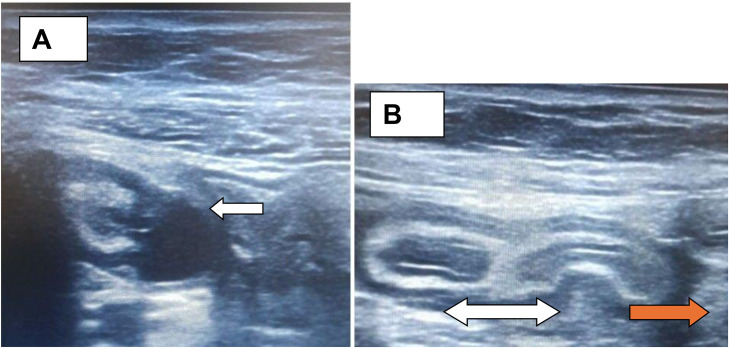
Representative ultrasound images in the transverse (A) and long-axis (B) planes demonstrate an enlarged appendix with focal outpouchings of the appendiceal wall, containing anechoic fluid with posterior acoustic enhancement, consistent with diverticula (arrow). The long-axis view shows appendix (double arrow head) with minimal periappendiceal inflammation (red arrow).
